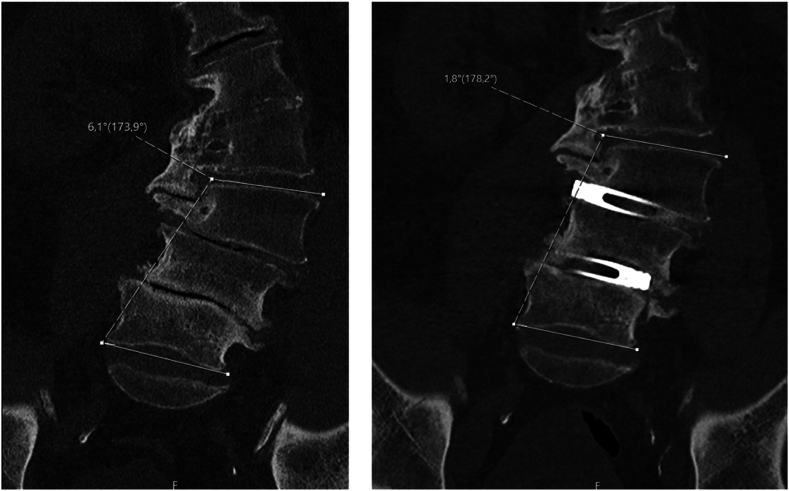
Preoperative (left) and postoperative (right) CT scans of the lumbar spine in coronal view including measurement of the Cobb angle.
